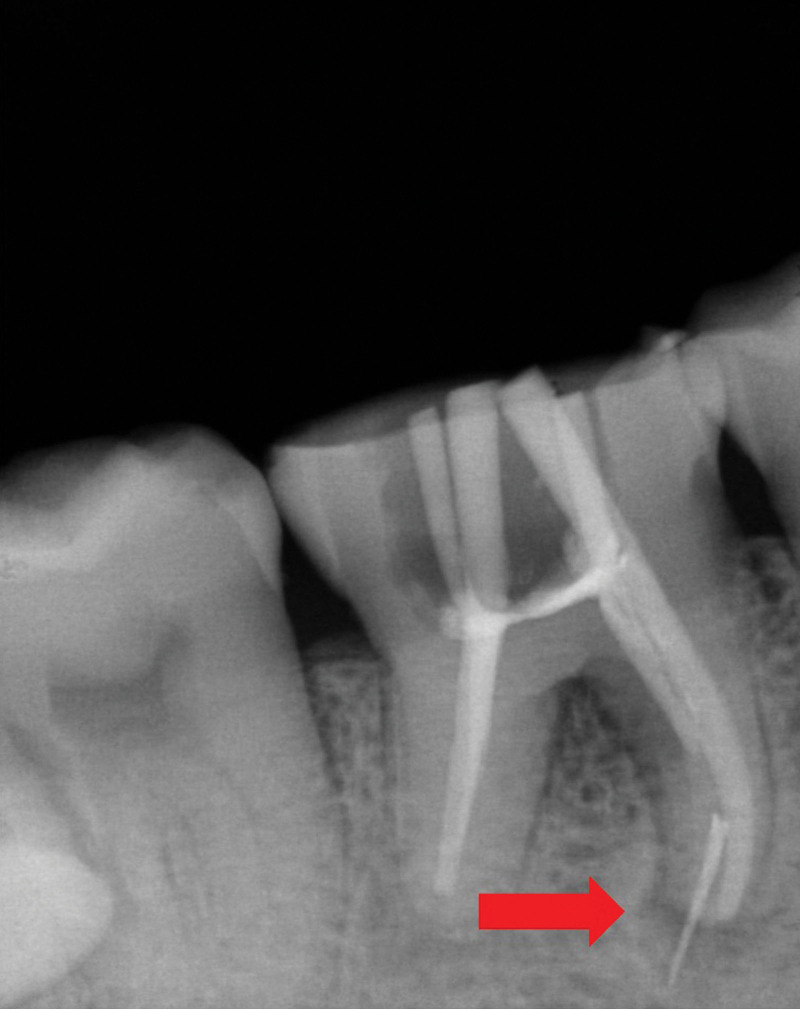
Periapical radiograph showing the separated instrument extending beyond the root apex.
